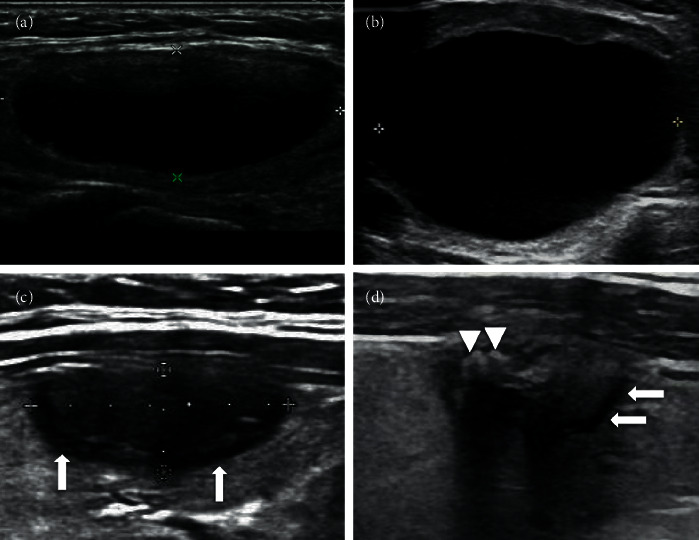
(a) A 44-year-old female was found a thyroid cystic nodule in a health examination, and it was classified as TI-RADS 2. (b) 1.5 years later, the patient felt neck pain, and also a mass with palpation. Ultrasound examination showed that the cyst was enlarged with the maximum diameter 40 mm, and dense punctate echoes could be seen in the capsule cavity, which was considered as hemorrhage in the cyst. (c) 2 years later, ultrasound showed that the nodule shrank and became solid inside, and the maximum diameter of the nodule was 16 mm. Arrows show a hypoechoic halo around nodules, and the margins of nodule were still clear. (d) 3 years later, the nodule was even smaller, and the hypoechoic halo around the nodule was still visible (arrows), but the margin became blurred. The triangle pointed to coarse calcifications, suggesting that necrotic substances exist for a long time. Echo attenuation behind the nodule suggested that fibrosis in the nodule might be serious.
